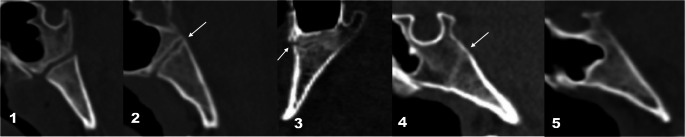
CT-based staging of spheno-occipital synchondrosis (SOS) ossification with arrow-guided landmarks. Representative sagittal CT images demonstrate the five-stage classification of SOS ossification according to the method adapted from Bassed et al. [18]. Arrows indicate the key morphological features used for stage assignment
